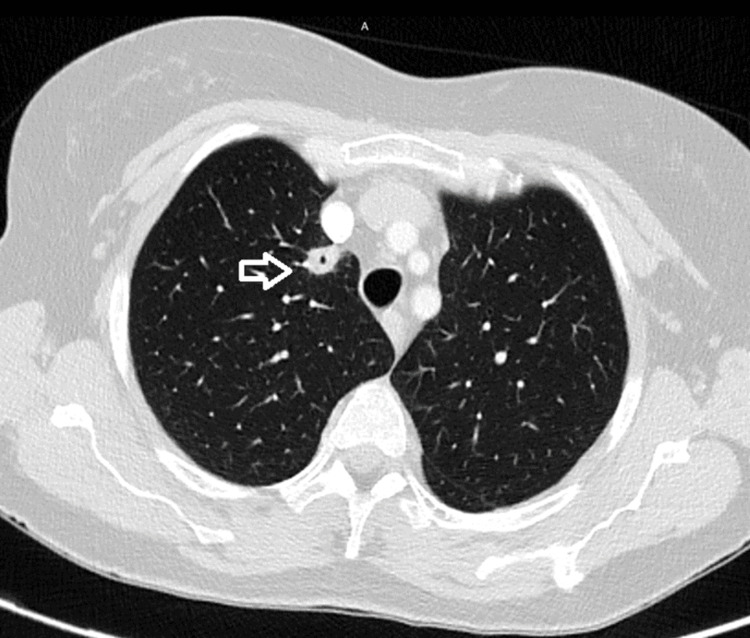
Axial CT imaging of the lung showing a cavitating lesion in the right upper lobe (white arrow).
